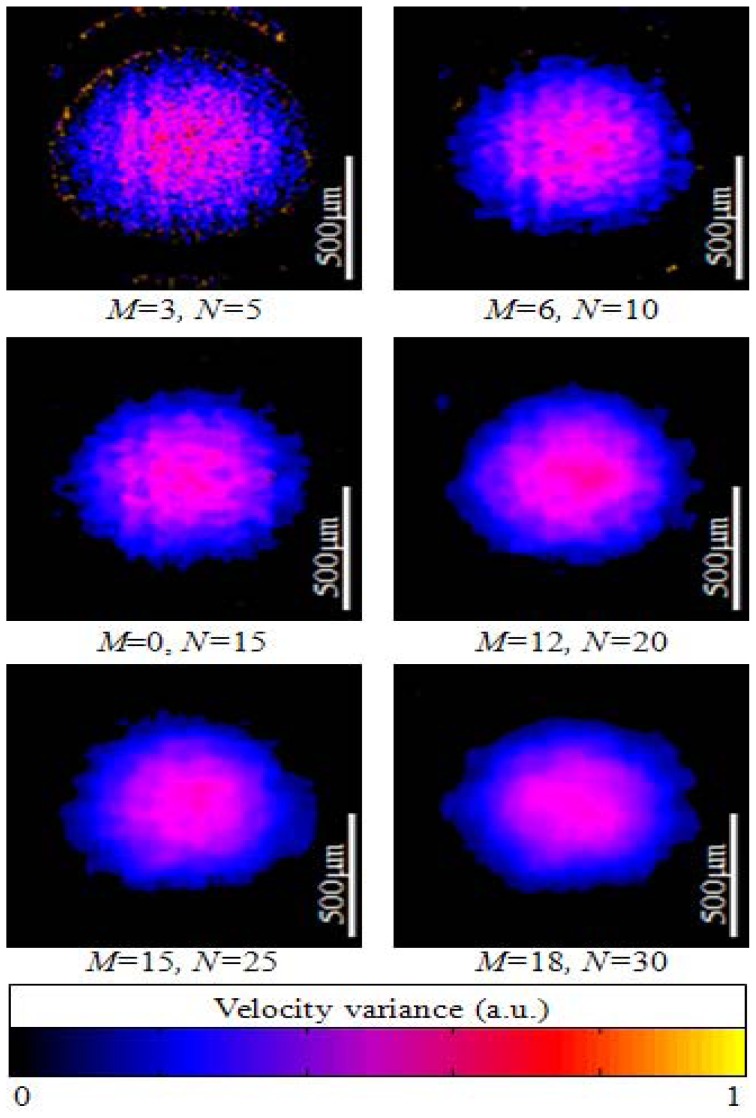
B-scan ODT images of flowing particles in a capillary tube with different averaging window sizes. Doppler angle: 80°. Flow velocity: 80 mm/s.
